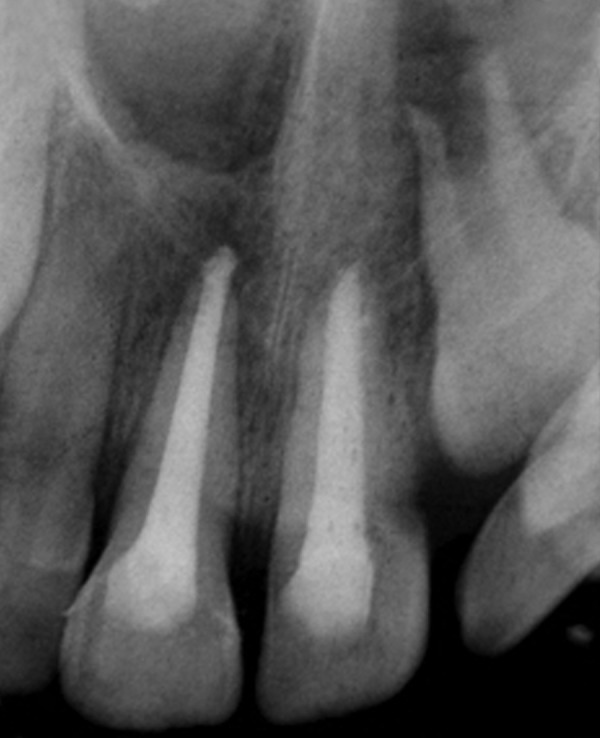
Postoperative intraoral periapical radiograph (case 5)
